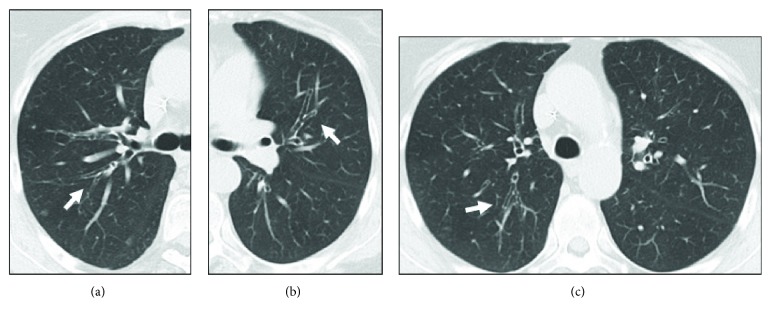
Multiple bilateral bronchiectasis in a 72-year-old woman with CD (arrows). Axial HRCT scans (a, b, c).
